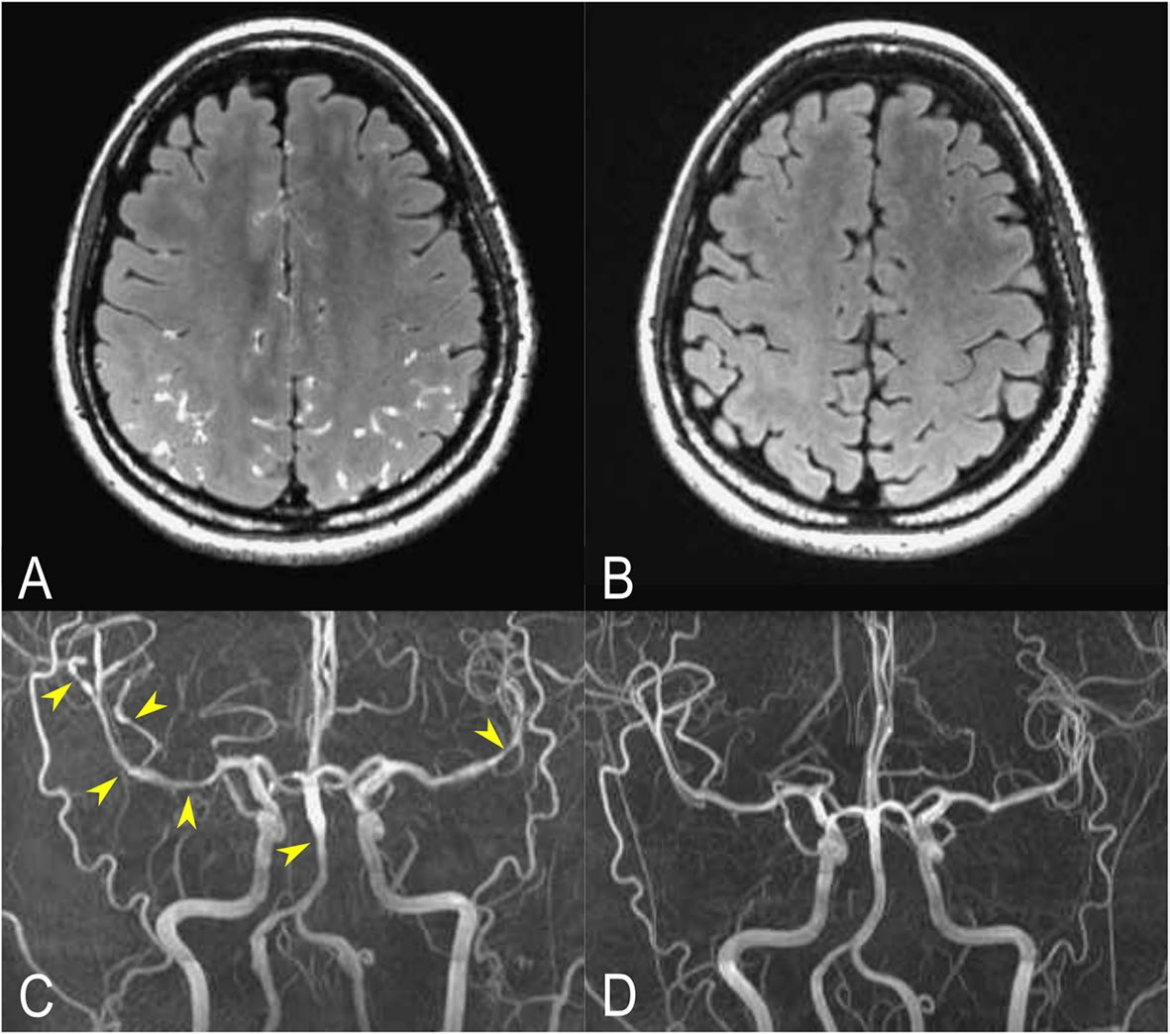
Segmentation of Blood-Brain Barrier Disruption in Patients with Reversible Cerebral Vasoconstriction Syndrome.  A  BBB disruption demonstrated by 3-dimensional contrast-enhanced T2-weighted-Fluid-Attenuated Inversion Recovery (FLAIR) image. B  Resolution of BBB disruption at three-month follow-up. C Vasoconstrictions observed by magnetic resonance angiography. D  Recovery of vasoconstrictions at three-month follow-up
